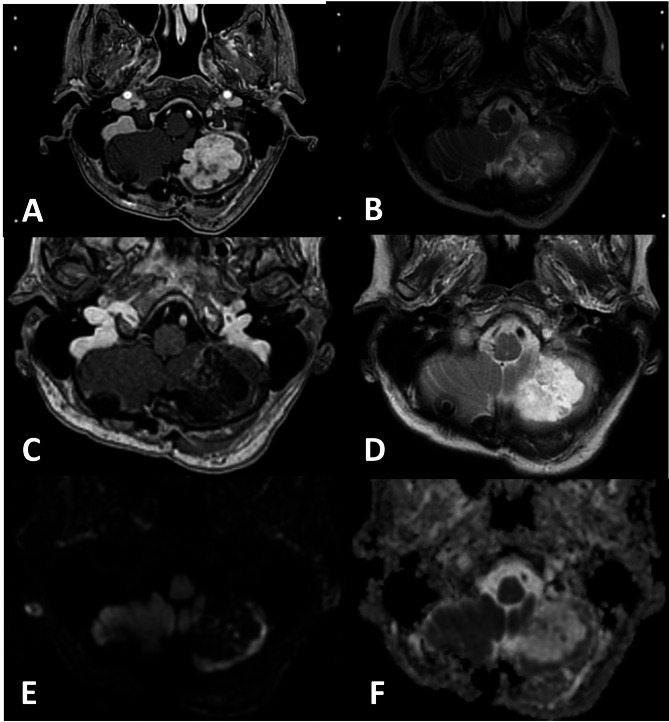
Examples of Adverse Radiation Events (ARE). A and B, stereotactic planning MRI corresponding to GKRS 1 demonstrates a lobulated, intensely enhancing, untreated viable metastasis in the left cerebellar hemisphere (a) with heterogeneous low signal on T2 (b). C to F: Follow-up MRI 13-months following RRR demonstrates no lesion size progression, with predominant markedly low signal on contrast enhanced T1 with mild streaky partial enhancement (c) and high signal on T2 (d), with no restricted diffusion evidenced by low signal on Diffusion Weighted Imaging (DWI) (e) and no regions lower than normal brain on the Apparent Diffusion Coefficient (ADC) image (f) consistent with radiation necrosis / ARE
